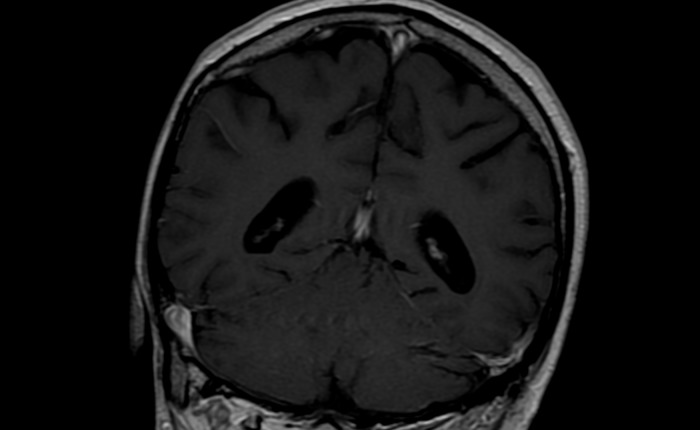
MRI brain of patient 1 shows resolution of lesion post treatment
